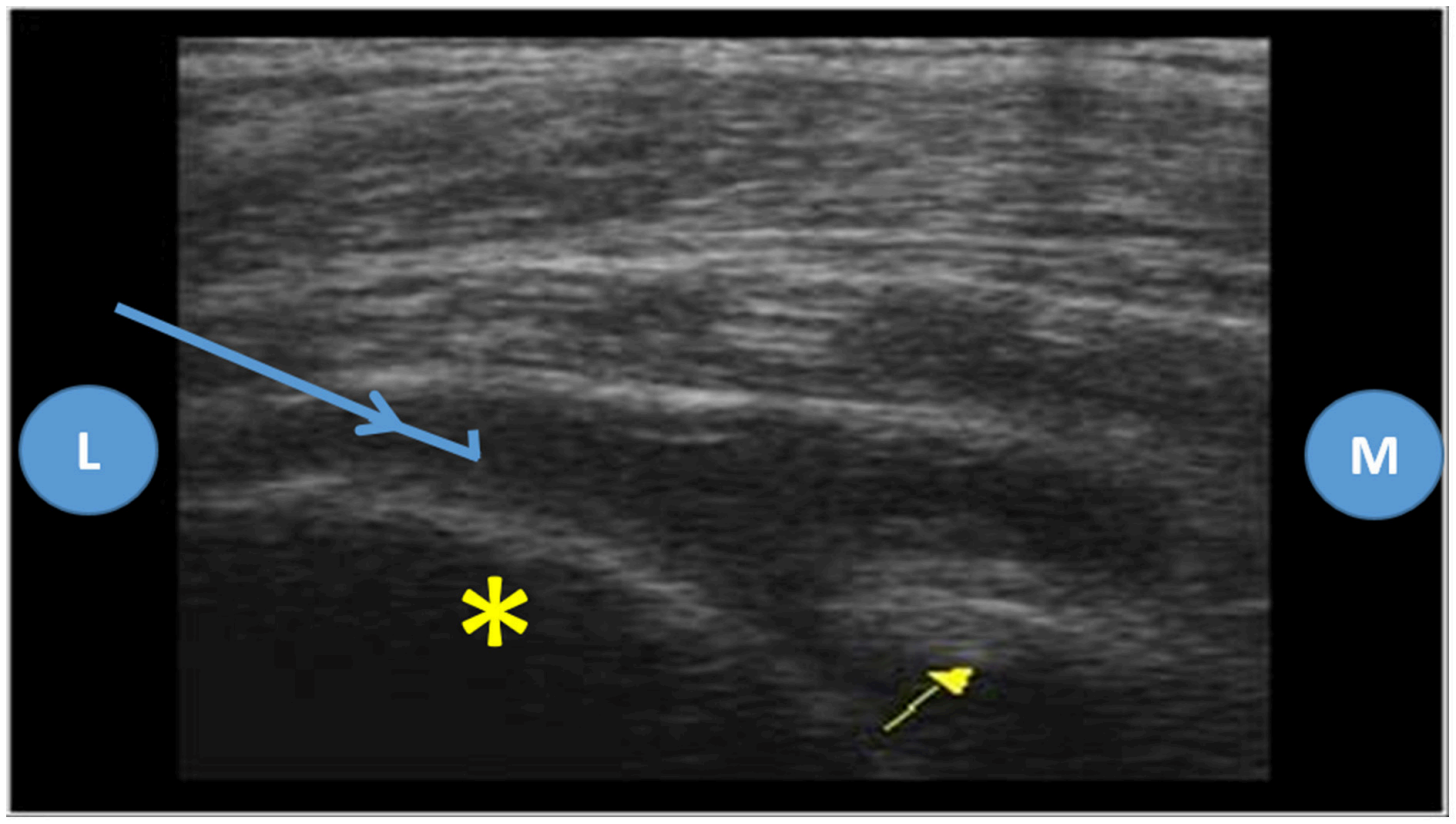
Posterior approach of the shoulder joint, ultrasound images. Probe placed on the long axis of the infraspinatus muscle, L, lateral; M, medial; Yellow asterisk—humeral head; Yellow arrow—glenoid; Blue arrow—biopsy needle trajectory.
